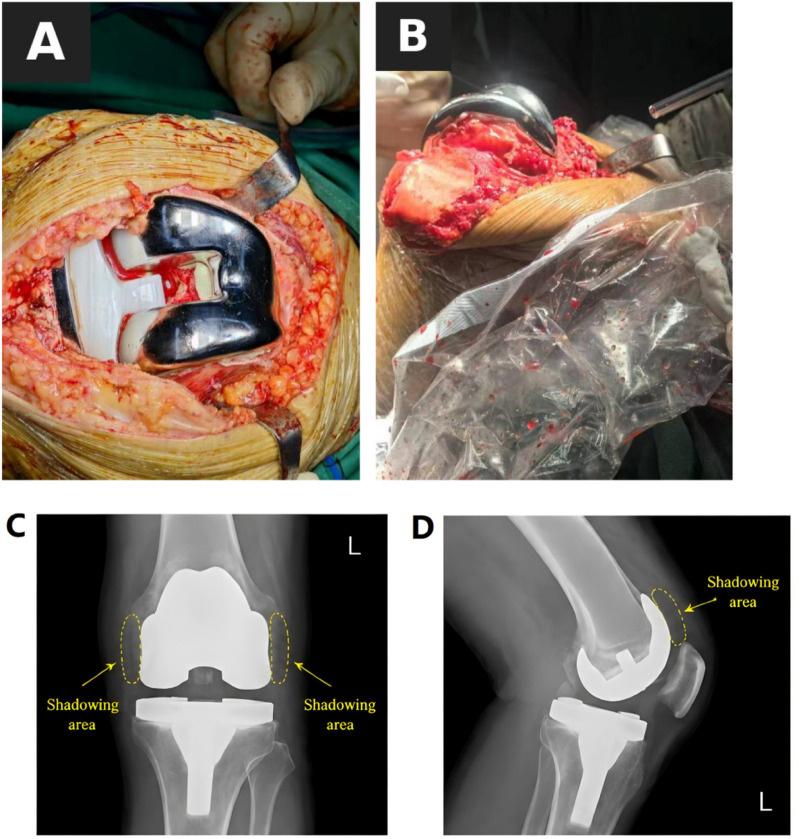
Representative intraoperative and radiographic findings of the uncovered distal femoral region. (A,B) Intraoperative views showing the uncovered distal femoral condylar region after trial component implantation. (C,D) Annotated postoperative anteroposterior and lateral radiographs showing focal reduction in bone density or subtle radiolucency in the uncovered distal femoral region, indicated by arrows.
